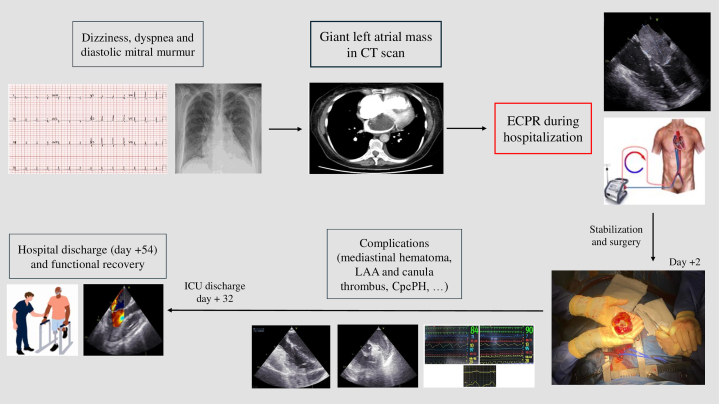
Summary of Case Presentation CpcPH = combined pre- and postcapillary pulmonary hypertension; CT = computed tomography; ECPR = extracorporeal cardiopulmonary resuscitation; ICU = intensive care unit; LAA = left atrial appendage.
